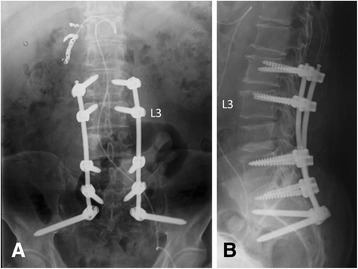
Postoperative day 3. a Posteroanterior view on postoperative radiography. b Lateral view on postoperative radiography
